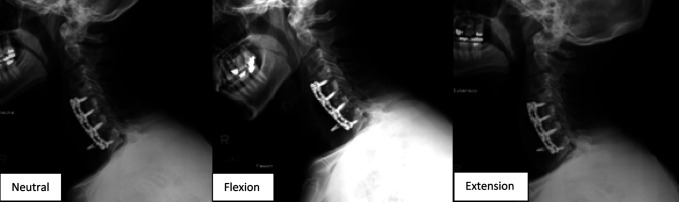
Cervical spine x-rays 12 years after ACDF demonstrating a completely loose and 180° rotated right C7 screw, along with gas anterior to the C4-7 ACDF plate. ACDF = anterior cervical diskectomy and fusion
